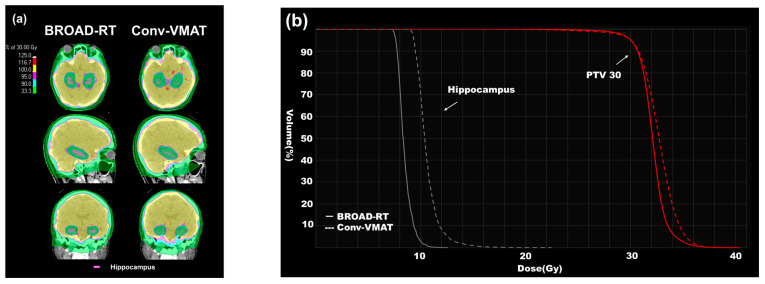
Differences in dose distribution (BROAD-RT vs. Conv-VMAT). (a) CT images displaying dose distribution of radiotherapy to HS-WBI (30 Gy in 10 fractions). The red line indicates the hippocampus. BROAD-RT achieved a sharp dose reduction in the hippocampal region compared to Conv-VMAT. (b) Dose–volume parameters of the PTV and hippocampus. Red line, PTV; white line, hippocampus. Compared to the Conv-VMAT plan, the BROAD-RT plan improved the important parameters of the hippocampus without deteriorating the PTV coverage. PTV, planning target volume; BROAD-RT, biaxially rotational dynamic radiation therapy; Conv, conventional; VMAT, volumetric modulated arc therapy; CT, computed tomography; HS-WBI, hippocampus-sparing whole brain irradiation.
